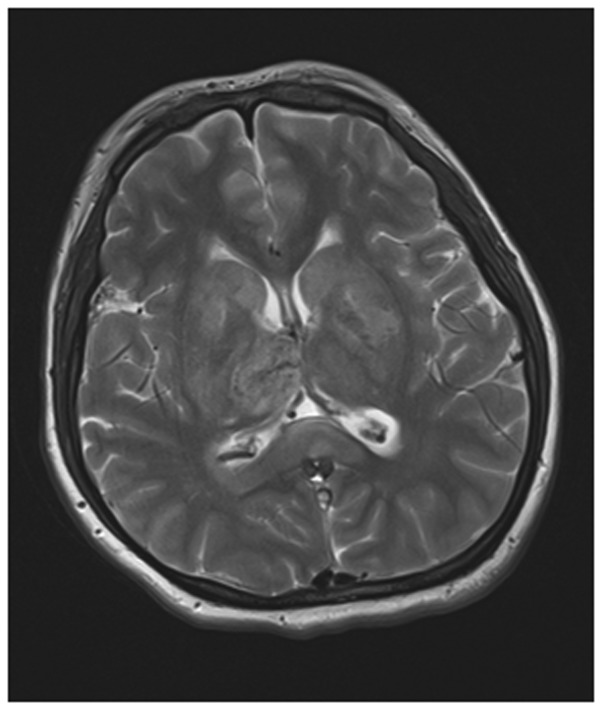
Magnetic resonance imaging (MRI) T2-weighted image displayed vein of Galen and basal ganglia with low signal intensities and corpus callosum splenium swelling.
